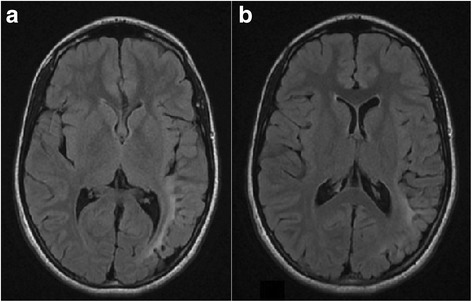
a, b Patient 4, brain magnetic resonance imaging, axial T2 FLAIR scans. An area of altered signal of the white matter in the temporal and parietal lobes can be observed, together with a reduction of the gray matter thickness in the left occipital sulcus (polymicrogiria)
